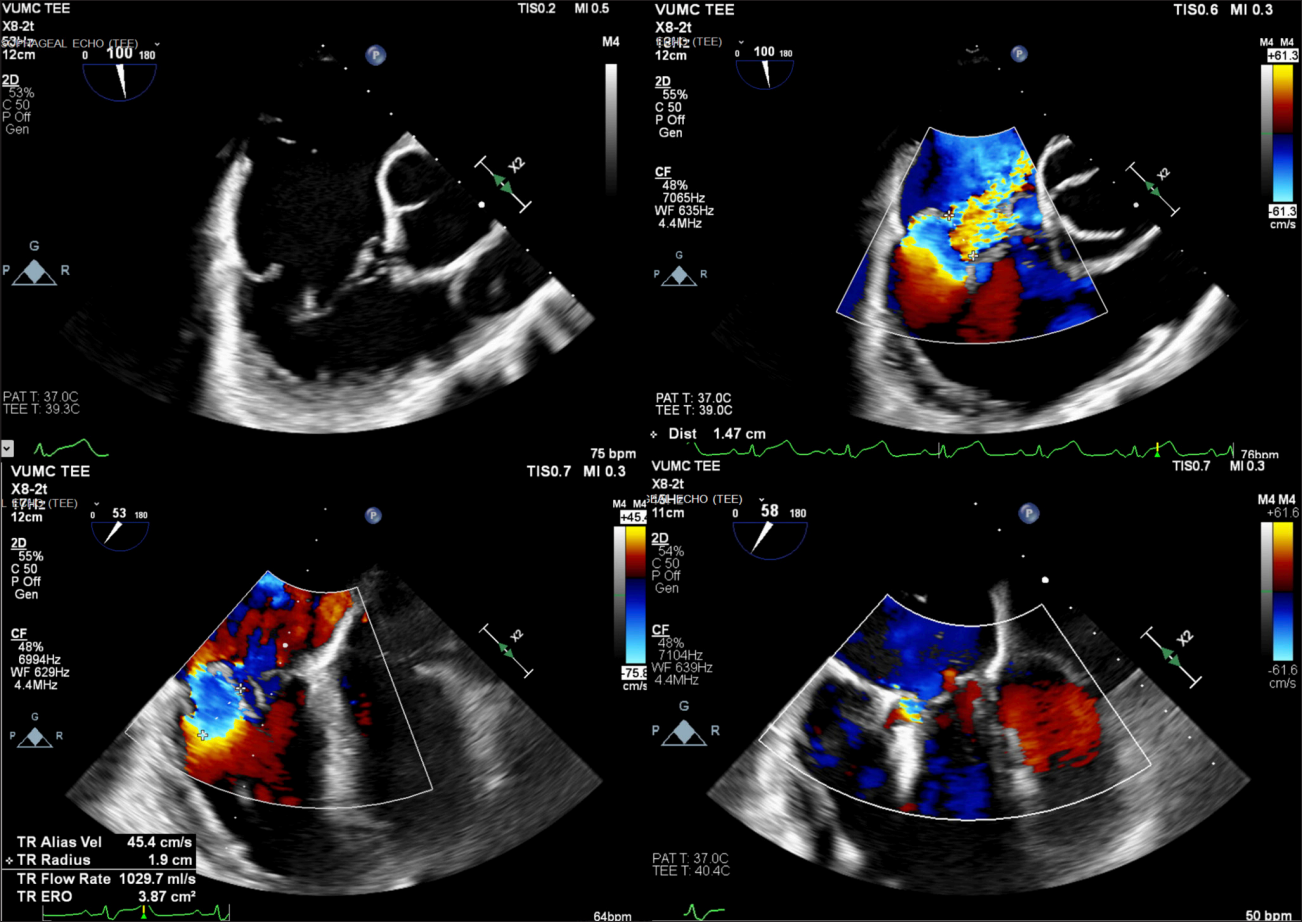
Transesophageal echocardiography intraprocedural image showing TriClip tricuspid valve repair. The tricuspid regurgitation went from torrential to trace after one anterior-posterior leaflet device and a second in the posterior-septal location.
